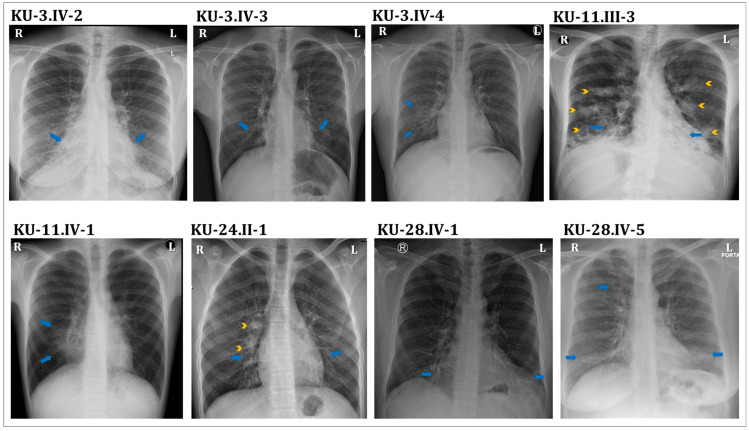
Radiological chest X-ray images for eight PCD individuals covered in this study. Chest X-ray of the KU-3.IV-2 patient showing mild diffuse bronchiectasis and patchy consolidations in bilateral lower zones, predominantly the retrocardiac regions (arrows), suggestive involvement of the right middle and the left lingular lobes. Chest X-ray of the KU-3.IV-3 patient showing dilated bronchi with “tram-track” appearance in the bilateral perihilar regions radiating into the lower zones (arrows). Chest X-ray of the KU-3.IV-4 patient showing subtle bronchiectatic changes with ill-defined hazy opacification in the medial right lower zone, suggestive of partial right middle lobe collapse (arrows). Chest X-ray of the KU-11.III-3 patient shows diffuse bilateral bronchiectasis with associated patchy opacities in all lung zones (arrowheads), representing secondary inflammatory changes (arrows). Chest X-ray of the KU-11.IV-1 patient showing central right perihilar bronchiectatic changes with an associated ill-defined opacity in the medial right lower zone causing obscuration of the right heart border, suggestive of complete right middle lobe collapse (arrows). Chest X-ray of the KU-24.II-1 patient showing dilated bronchi with “tram-track” appearance in the bilateral perihilar regions radiating into the lower zones (arrows). Small patches of consolidation are also seen in the right perihilar region (arrowheads), denoting infective changes. Chest X-ray of the KU-28.IV-1 patient showing mild diffuse bronchiectasis and patchy consolidations in bilateral lower zones, predominantly the retrocardiac regions (arrows), suggestive of involvement of the right middle and the left lingular lobes. Chest X-ray of the KU-28.IV-5 patient showing mild diffuse bronchiectasis and patchy consolidations in bilateral lower and the right upper zones (arrows). Situs solitus is seen in all patients.
